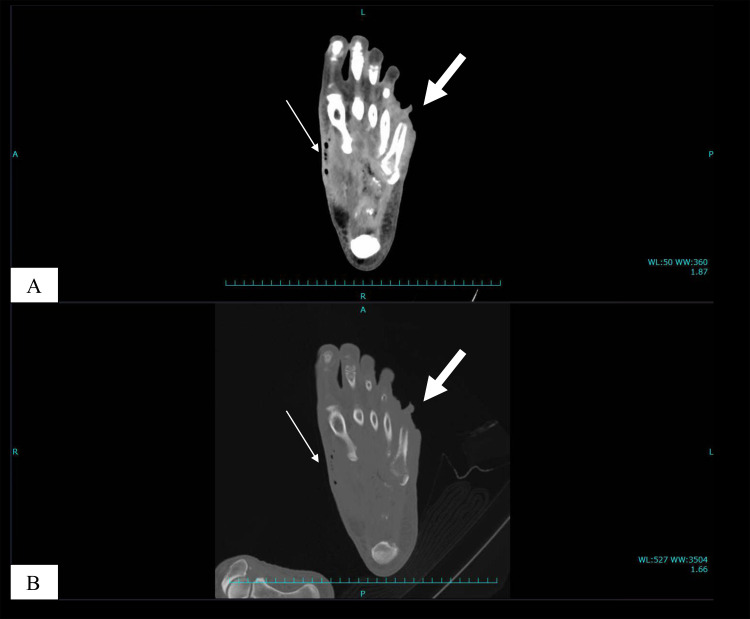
CT images of the injured site. (A & B) The proximal-distal phalangeal bones of the fifth toe show absent signals (thick white arrow). An abscess with gas was present in the plantar region (thin white arrow).
